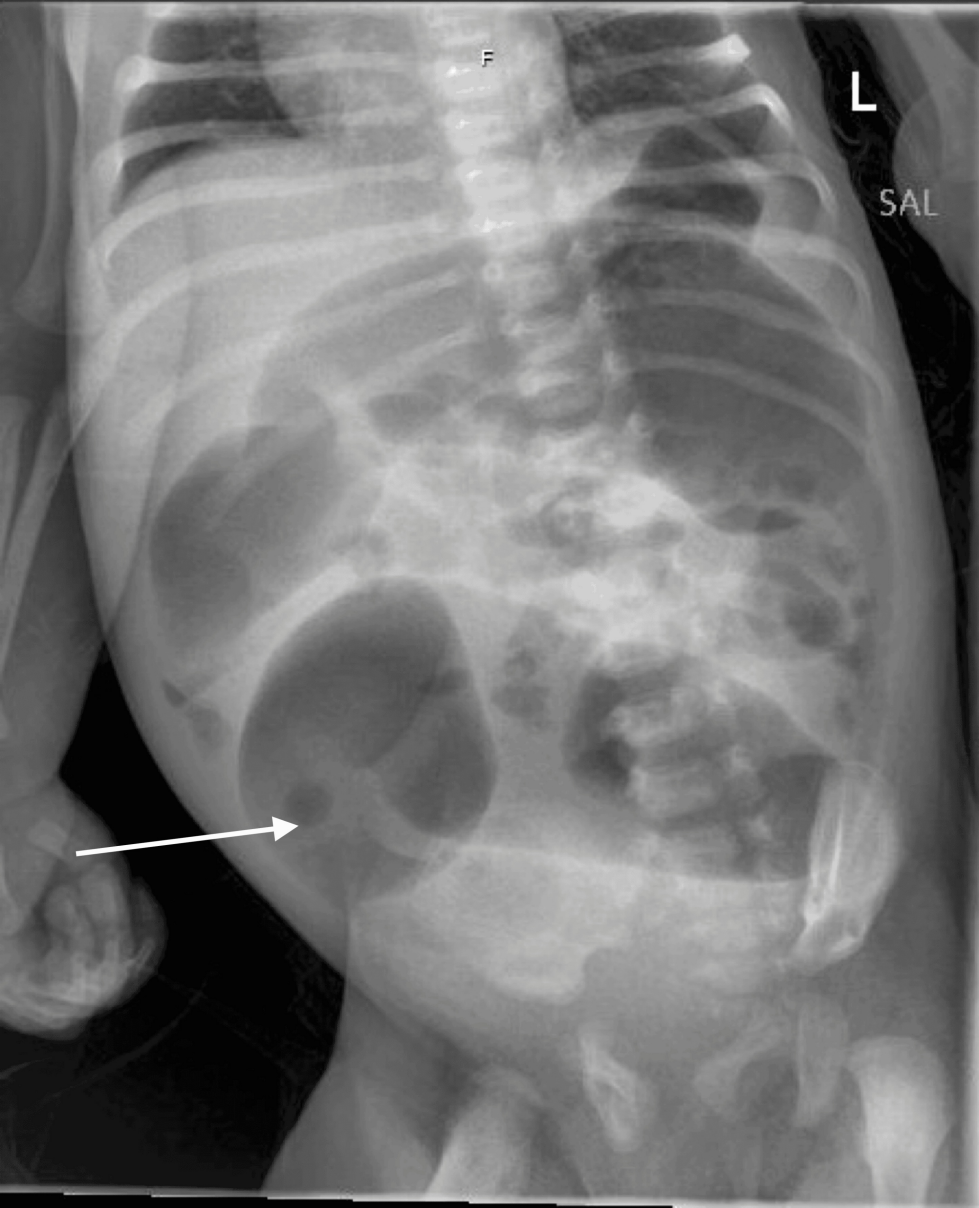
One-view X-ray abdomen taken on day 1 of the second admission The arrow points to a prominent air-filled loop of the bowel. Findings: A prominent air-filled loop of the bowel measuring 4.5 cm in diameter within the lower abdomen without evidence of rectal air. Loops of normal caliber small bowel are seen within the mid-abdomen. No evidence of bowel obstruction. The visualized osseous structures are normal.
